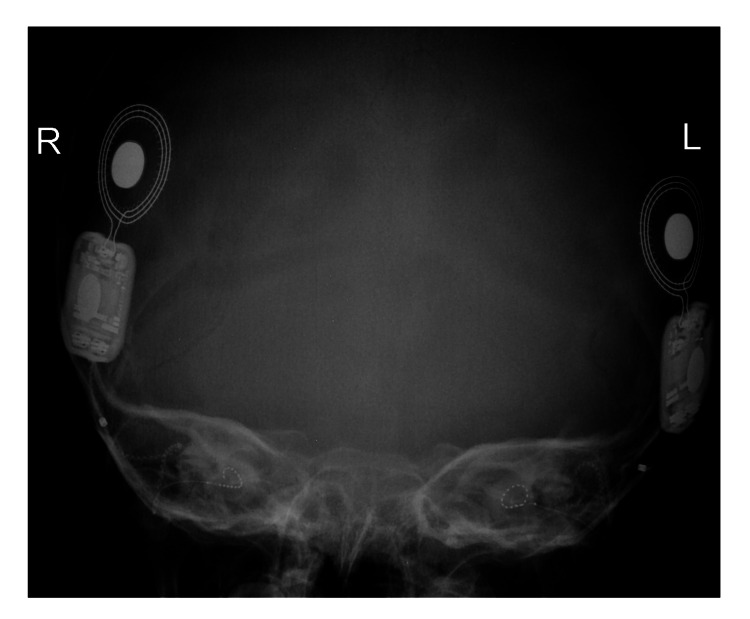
Postoperative x-ray temporal bone of Case 2 showing complete insertion of the cochlear implant electrode array at both ears
